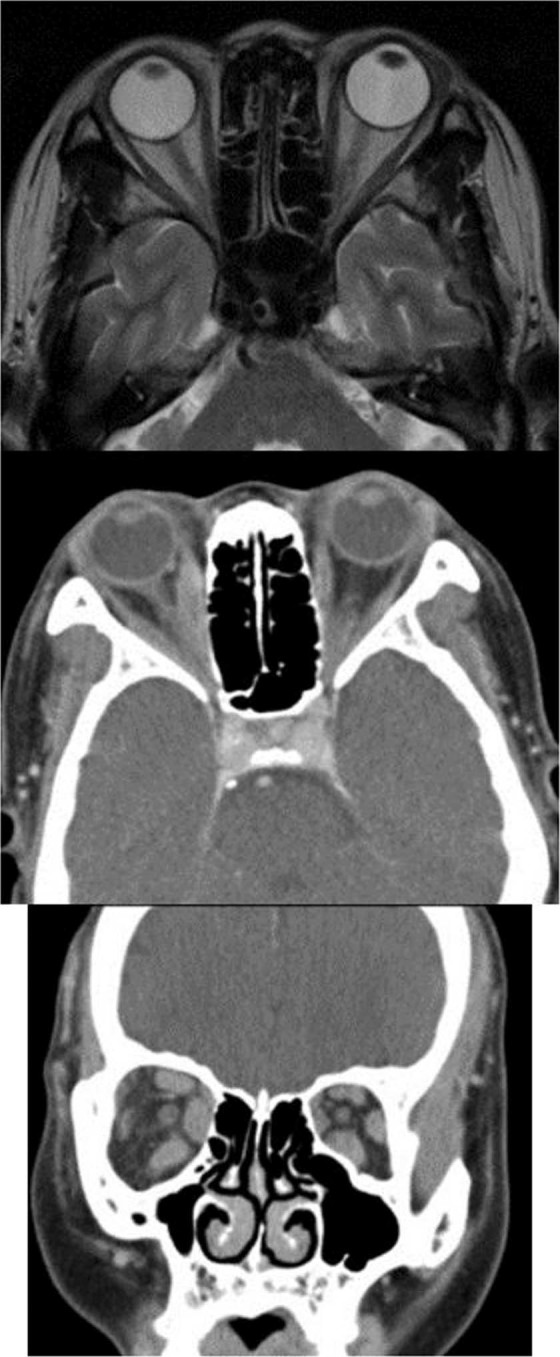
Change of extraocular muscle (EOM) in patient with thyroid autoantibodies during follow-up. The imaging scans of one patient who was positive for thyroid autoantibodies. (a) At first visit, he presented 6 prism diopters of horizontal deviation angle. There was no EOM limitation and thyroid function was normal. MRI presented no abnormal lesion in brain and normal thickness of extraocular muscle. (b,c) After 42 months of follow-up, diplopia was getting worse and patient was newly diagnosed with Graves’ disease. The EOM was significantly thickened. Change of horizontal deviation angle was 11 PD and 12PD of vertical deviation was newly presented.
